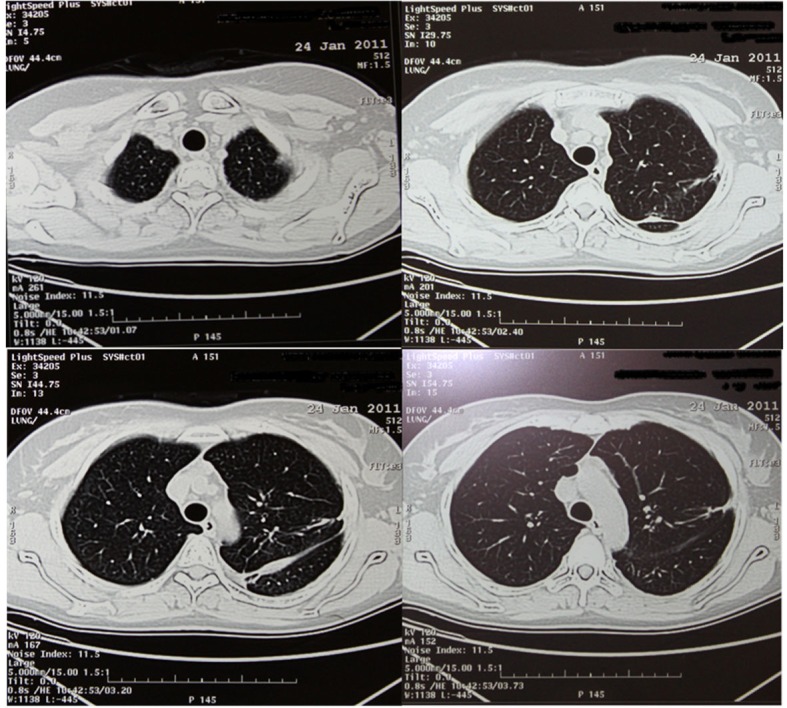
CT scan at follow up (4 months postoperatively).
